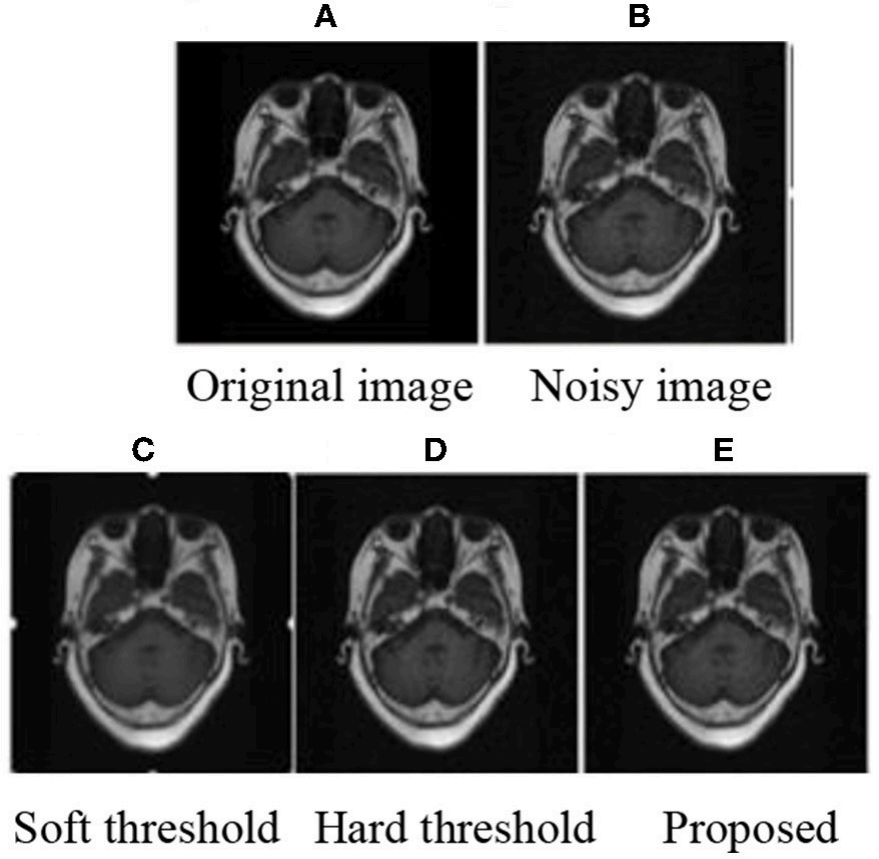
MRI 2 of subjective results.
